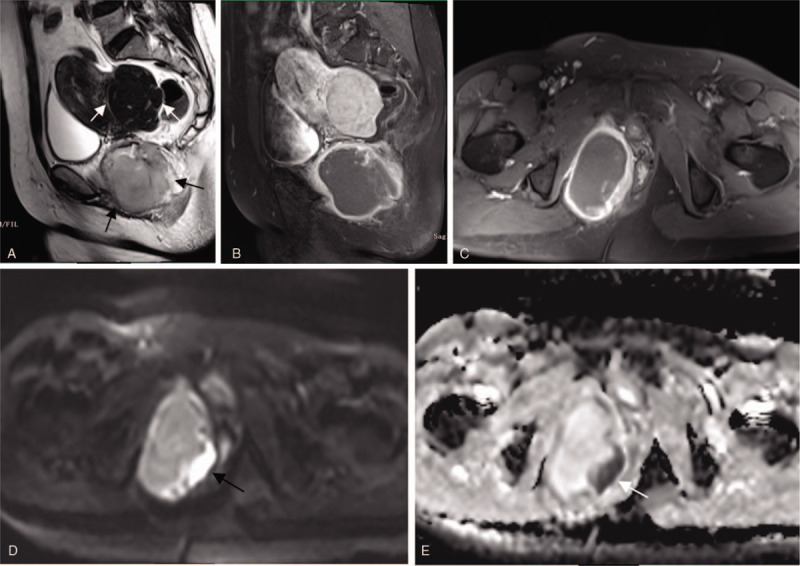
MR examination of the pelvis. (A) Sagittal T2-weighted image showed a 7.5 × 4.9 cm solid and cystic mass with obscure margin (black arrow) and a 6.7 × 4.8 cm smooth, well-defined mass (white arrow). (B) Sagittal T1-weighted-enhanced image showed that the uterine tumor appears as heterogeneous enhancement, in the posterior uterine wall. (C) Axial T1-weighted-enhanced image showed that the vaginal tumor's edge appears as marked enhancement, in the ischiorectal fossa adjacent to the right lateral vaginal wall. (D) Axial diffusion-weighted image and (E) apparent diffusion coefficient image showed that the vaginal tumor's edge appears as restricted diffusion (black and white arrow).
